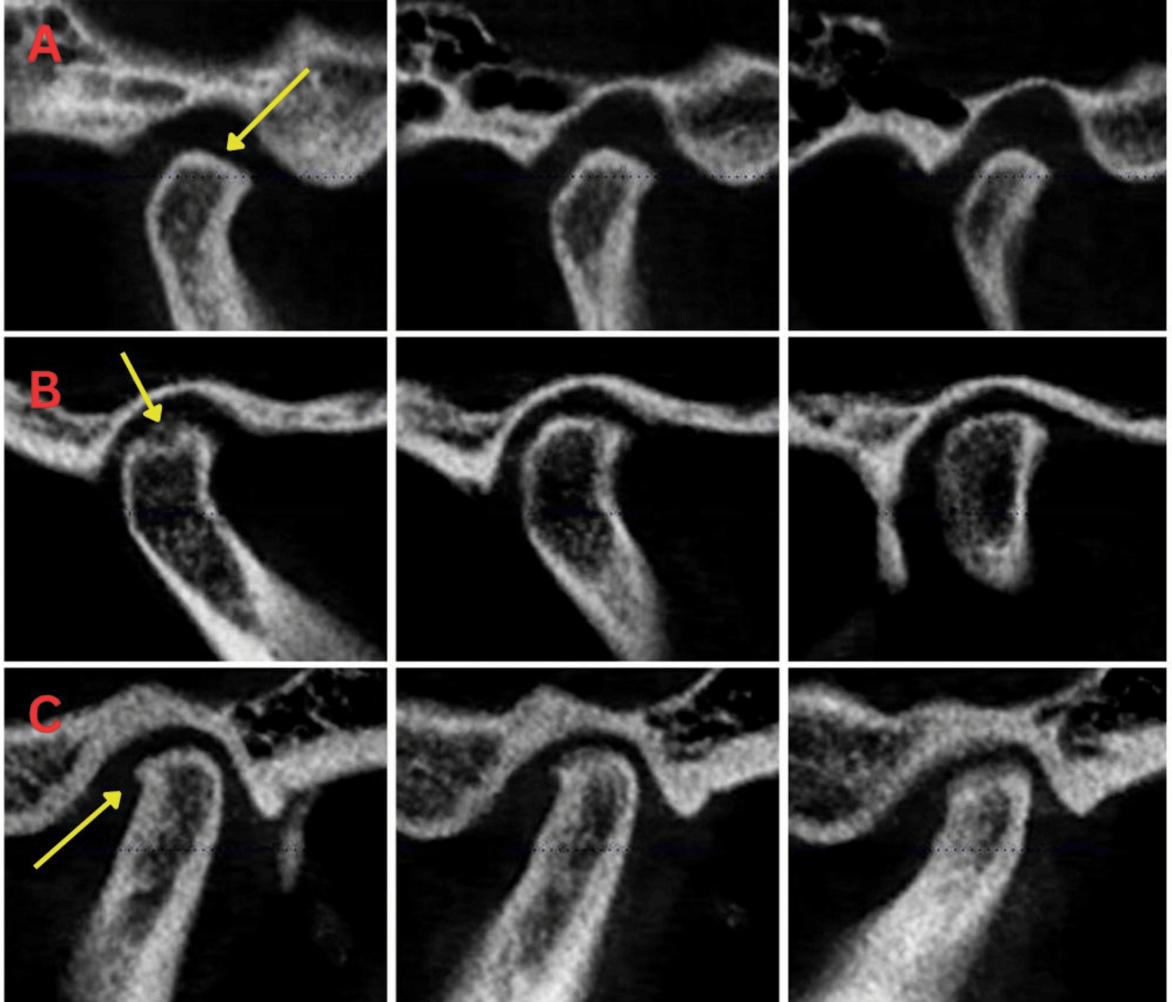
CBCT imaging showing TMJ grade 2. A: Moderate flattening of contours. B: Less than three erosions. C: Small osteophyte. CBCT: cone-beam computed tomography; TMJ: temporomandibular joint.
